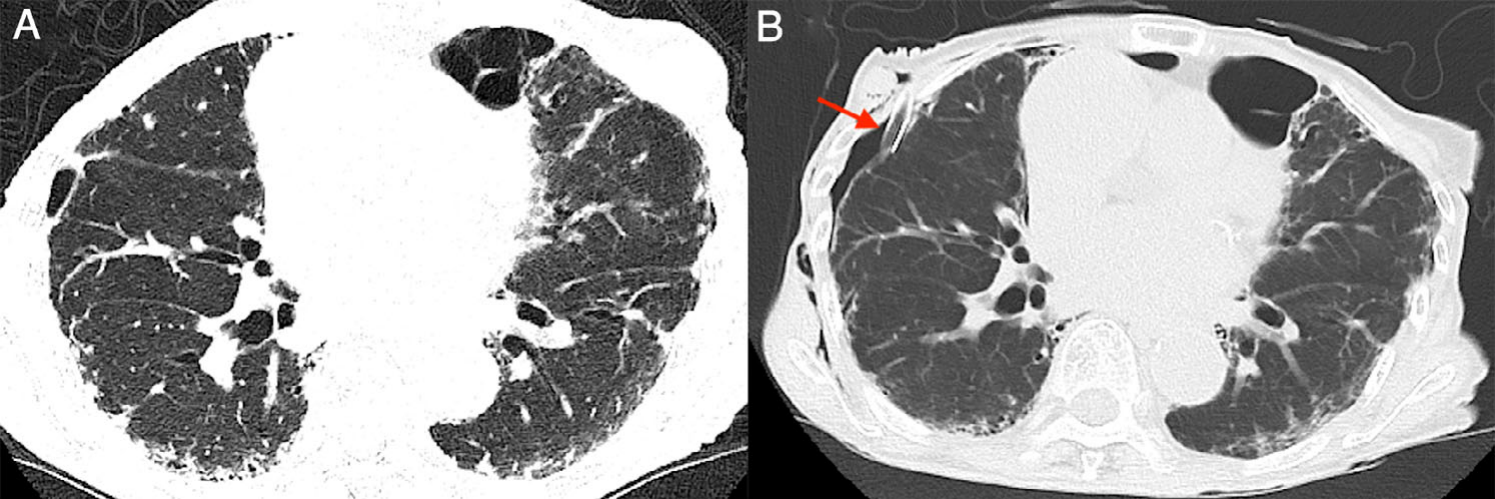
(A) Chest computed tomography showing subpleural interstitial shadows in the lungs. (B) A tube is inserted in the right thoracic cavity (red arrow), and mild subcutaneous emphysema is observed.
